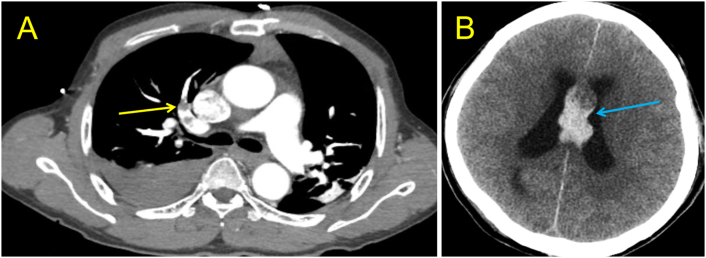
The CTA chest and CT head of patient 2 after thrombolytic therapy. A: after thrombolytic therapy, CTA shows right pulmonary artery filling defect (yellow arrow); B: On day 3 after thrombolytic therapy, CT showed septum pellucidum hemorrhage and broke into bilateral ventricles (blue arrow), brain edema with herniation formation.
